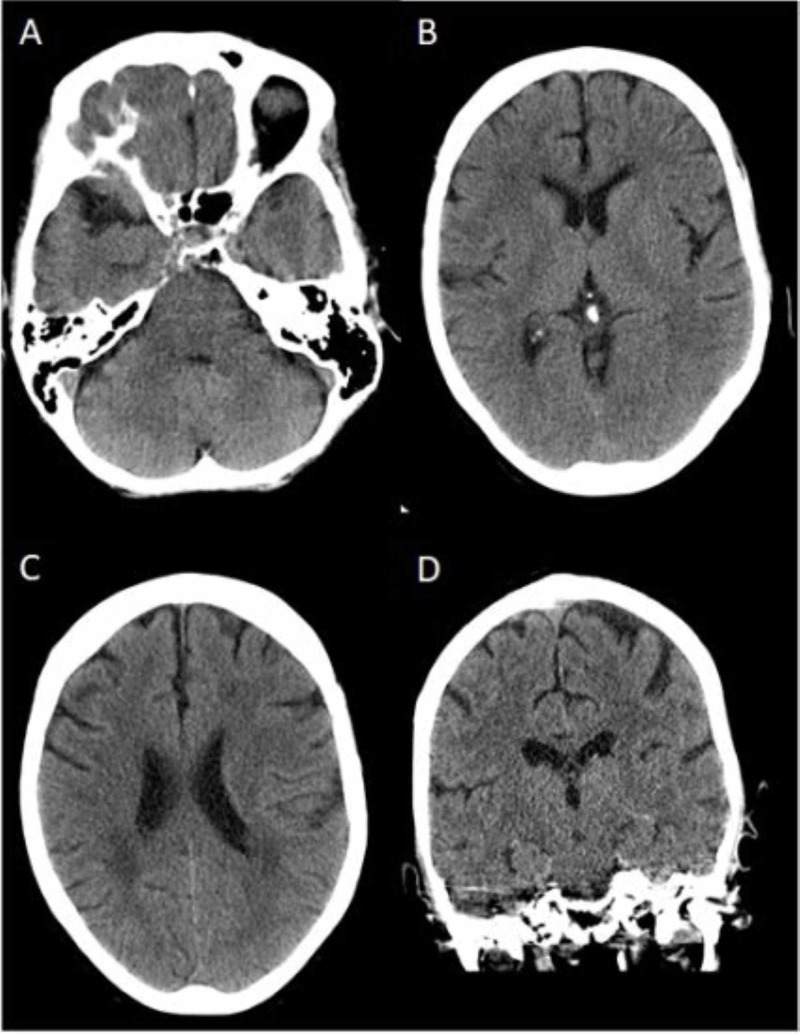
CT of the head without intravenous contrast A,B,C: axial view; D: coronal view No acute intracranial process is seen CT: computed tomography
